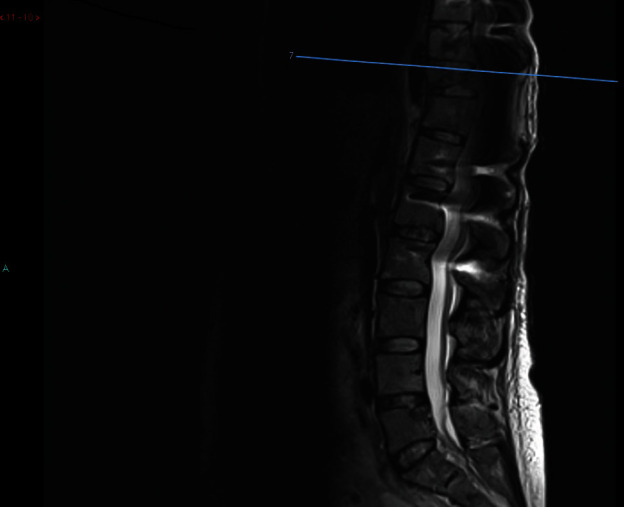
Postoperative MRI T2WI (sagittal image, showing the level of surgery).
